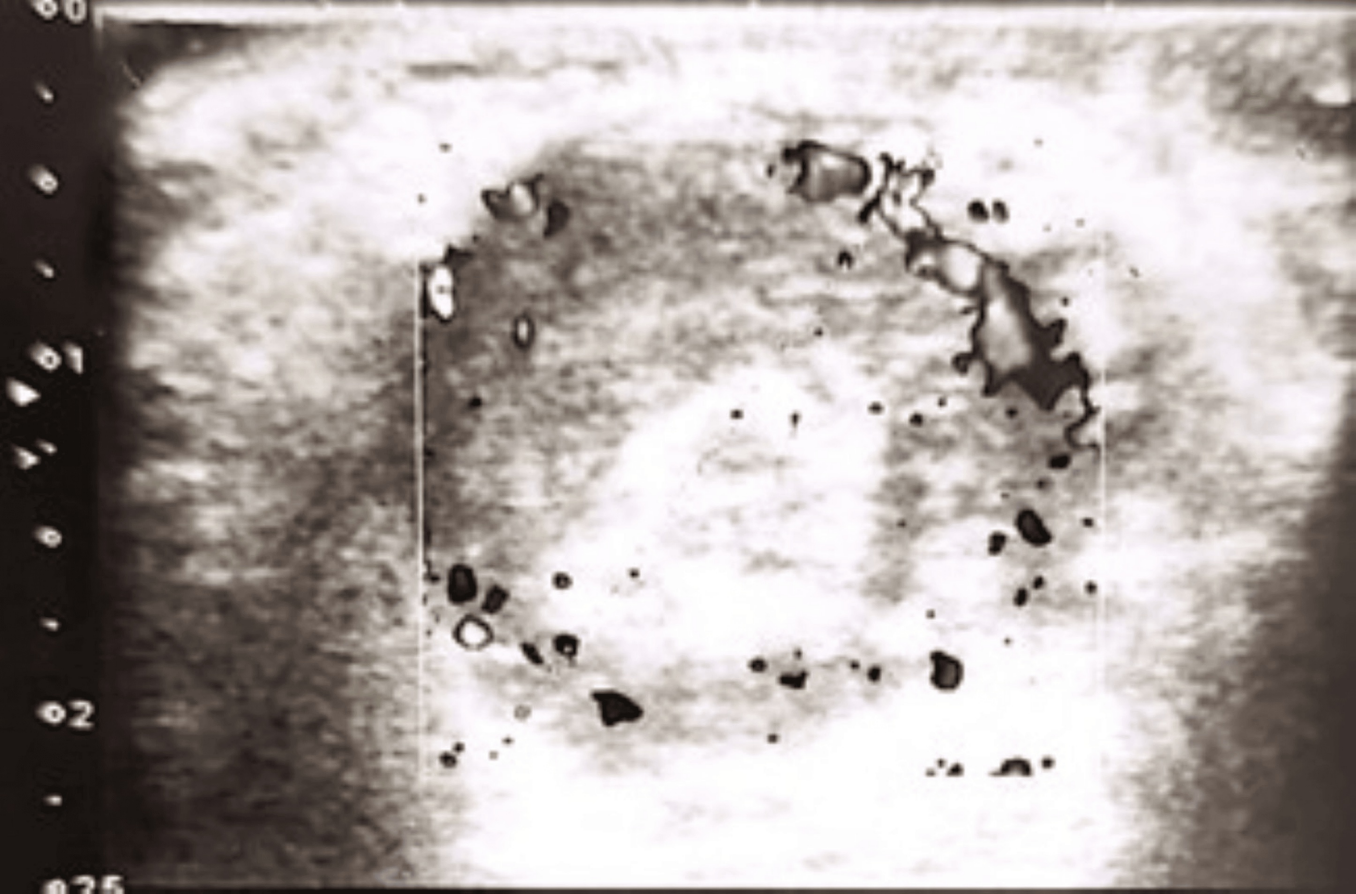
Doppler ultrasound showing reduced central blood flow with preservation of peripheral blood flow in right testis.
